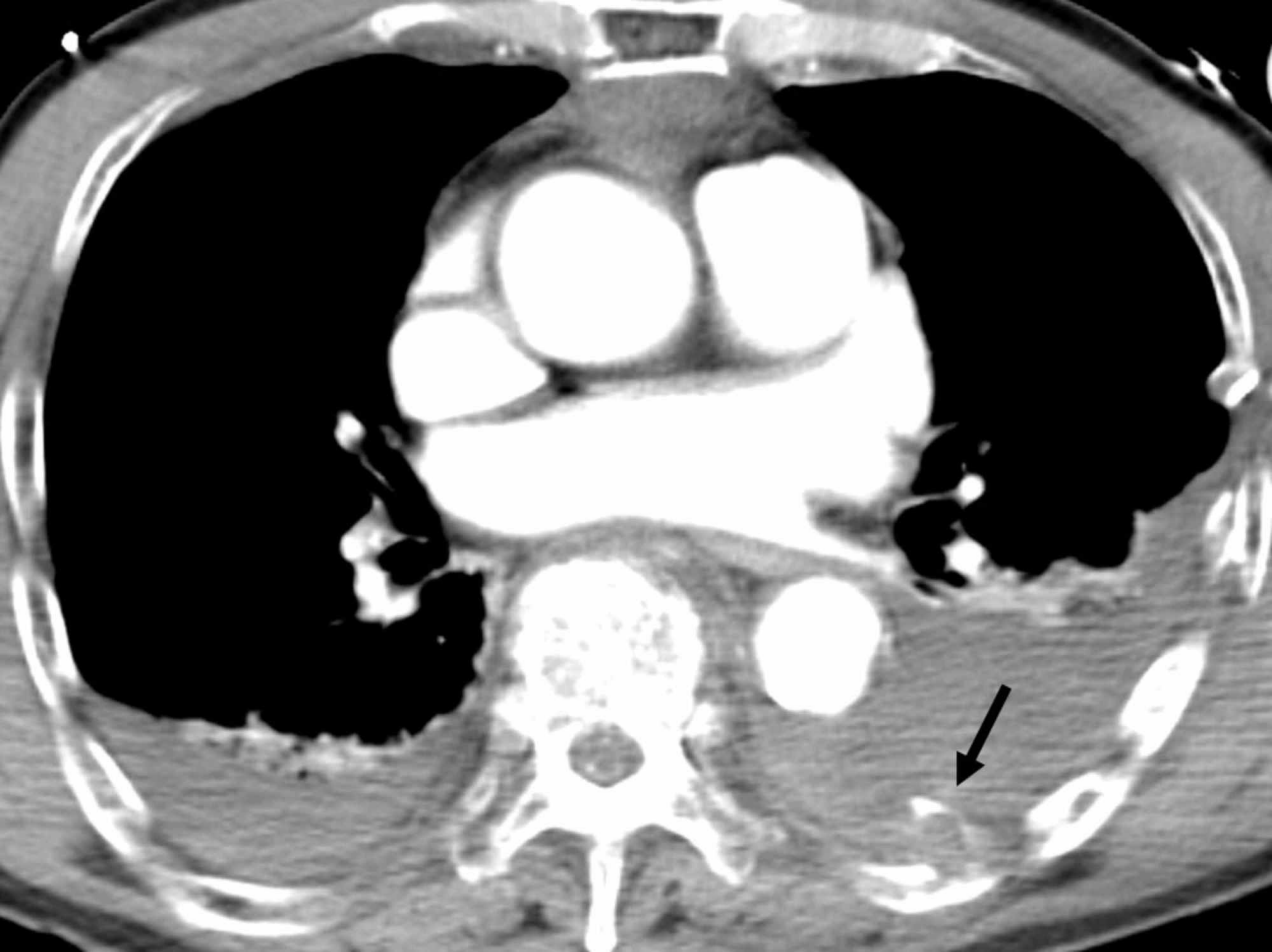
Computed tomography (CT) on arrival CT revealed multiple left rib and sternal fractures with left-dominant bilateral hemothorax and anterior mediastinal hematoma. The figure shows extravasation of contrast medium (arrow).
